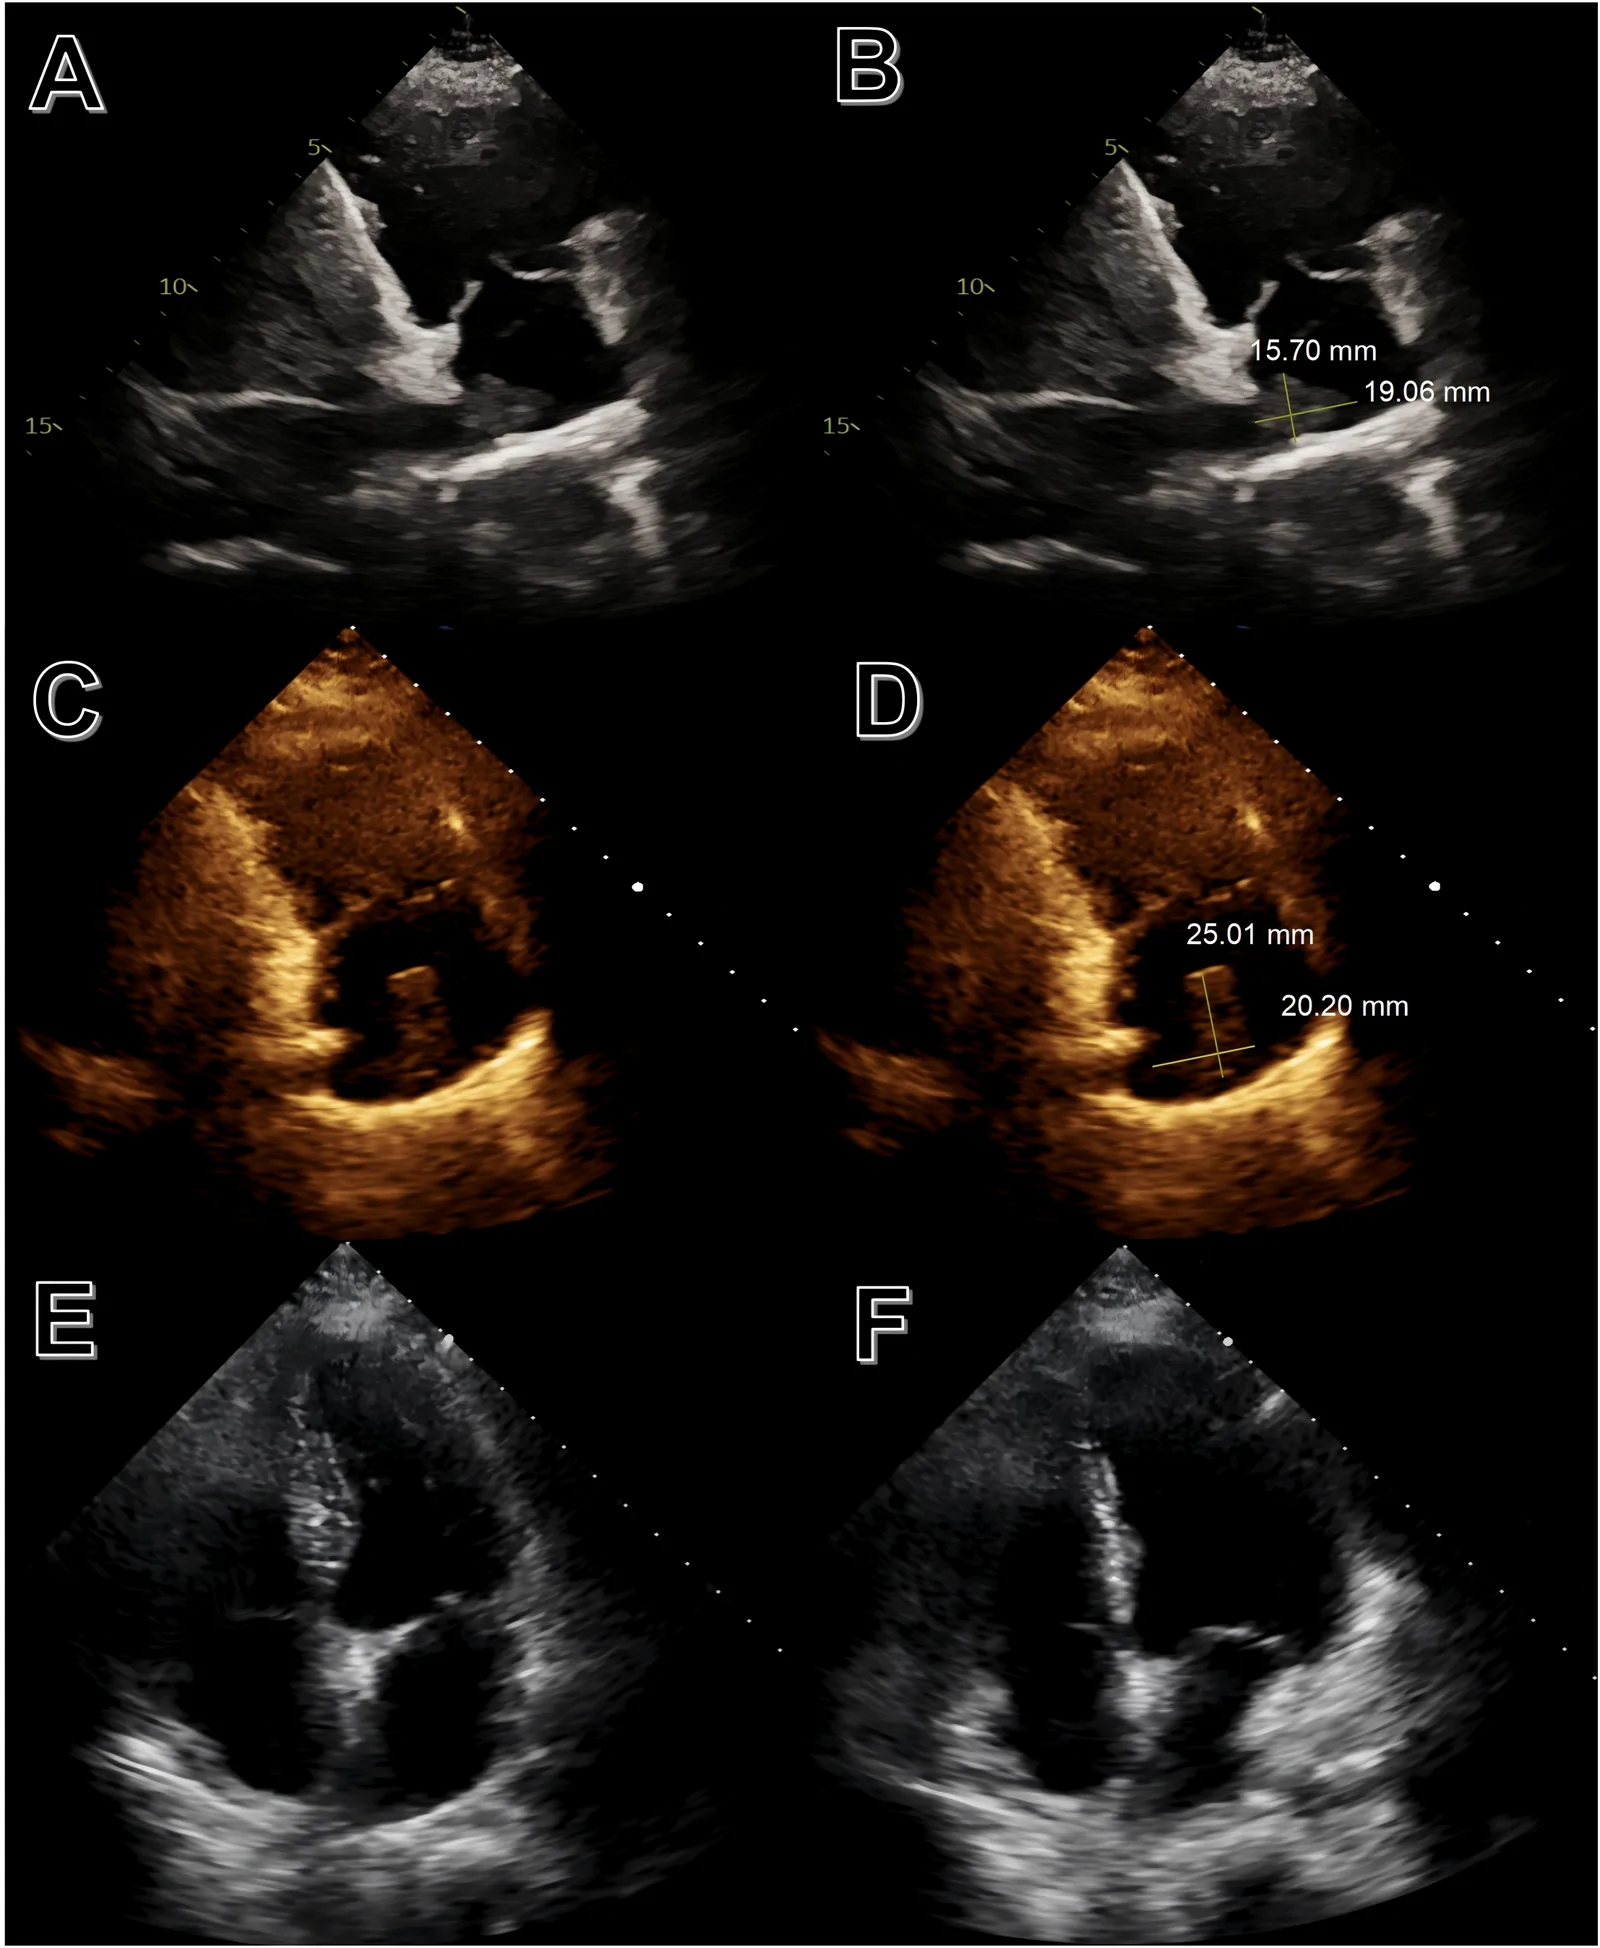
Serial echocardiographic findings. (A, B) echocardiographic images obtained on December 9, 2025. (C, D) contrast-enhanced echocardiographic images obtained on December 16, 2025. (E, F) echocardiographic images obtained on December 17, 2025.
